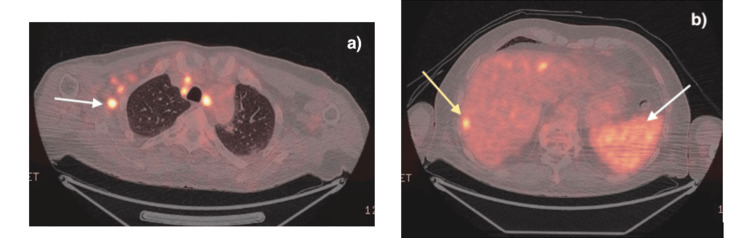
Positron emission tomography (PET) scan showing fludeoxyglucose (FDG) avid lymphadenopathy with hepatic and splenic uptake a: lymphadenopathy (white arrow); b: splenic uptake (white arrow), and hepatic uptake (yellow arrow).
